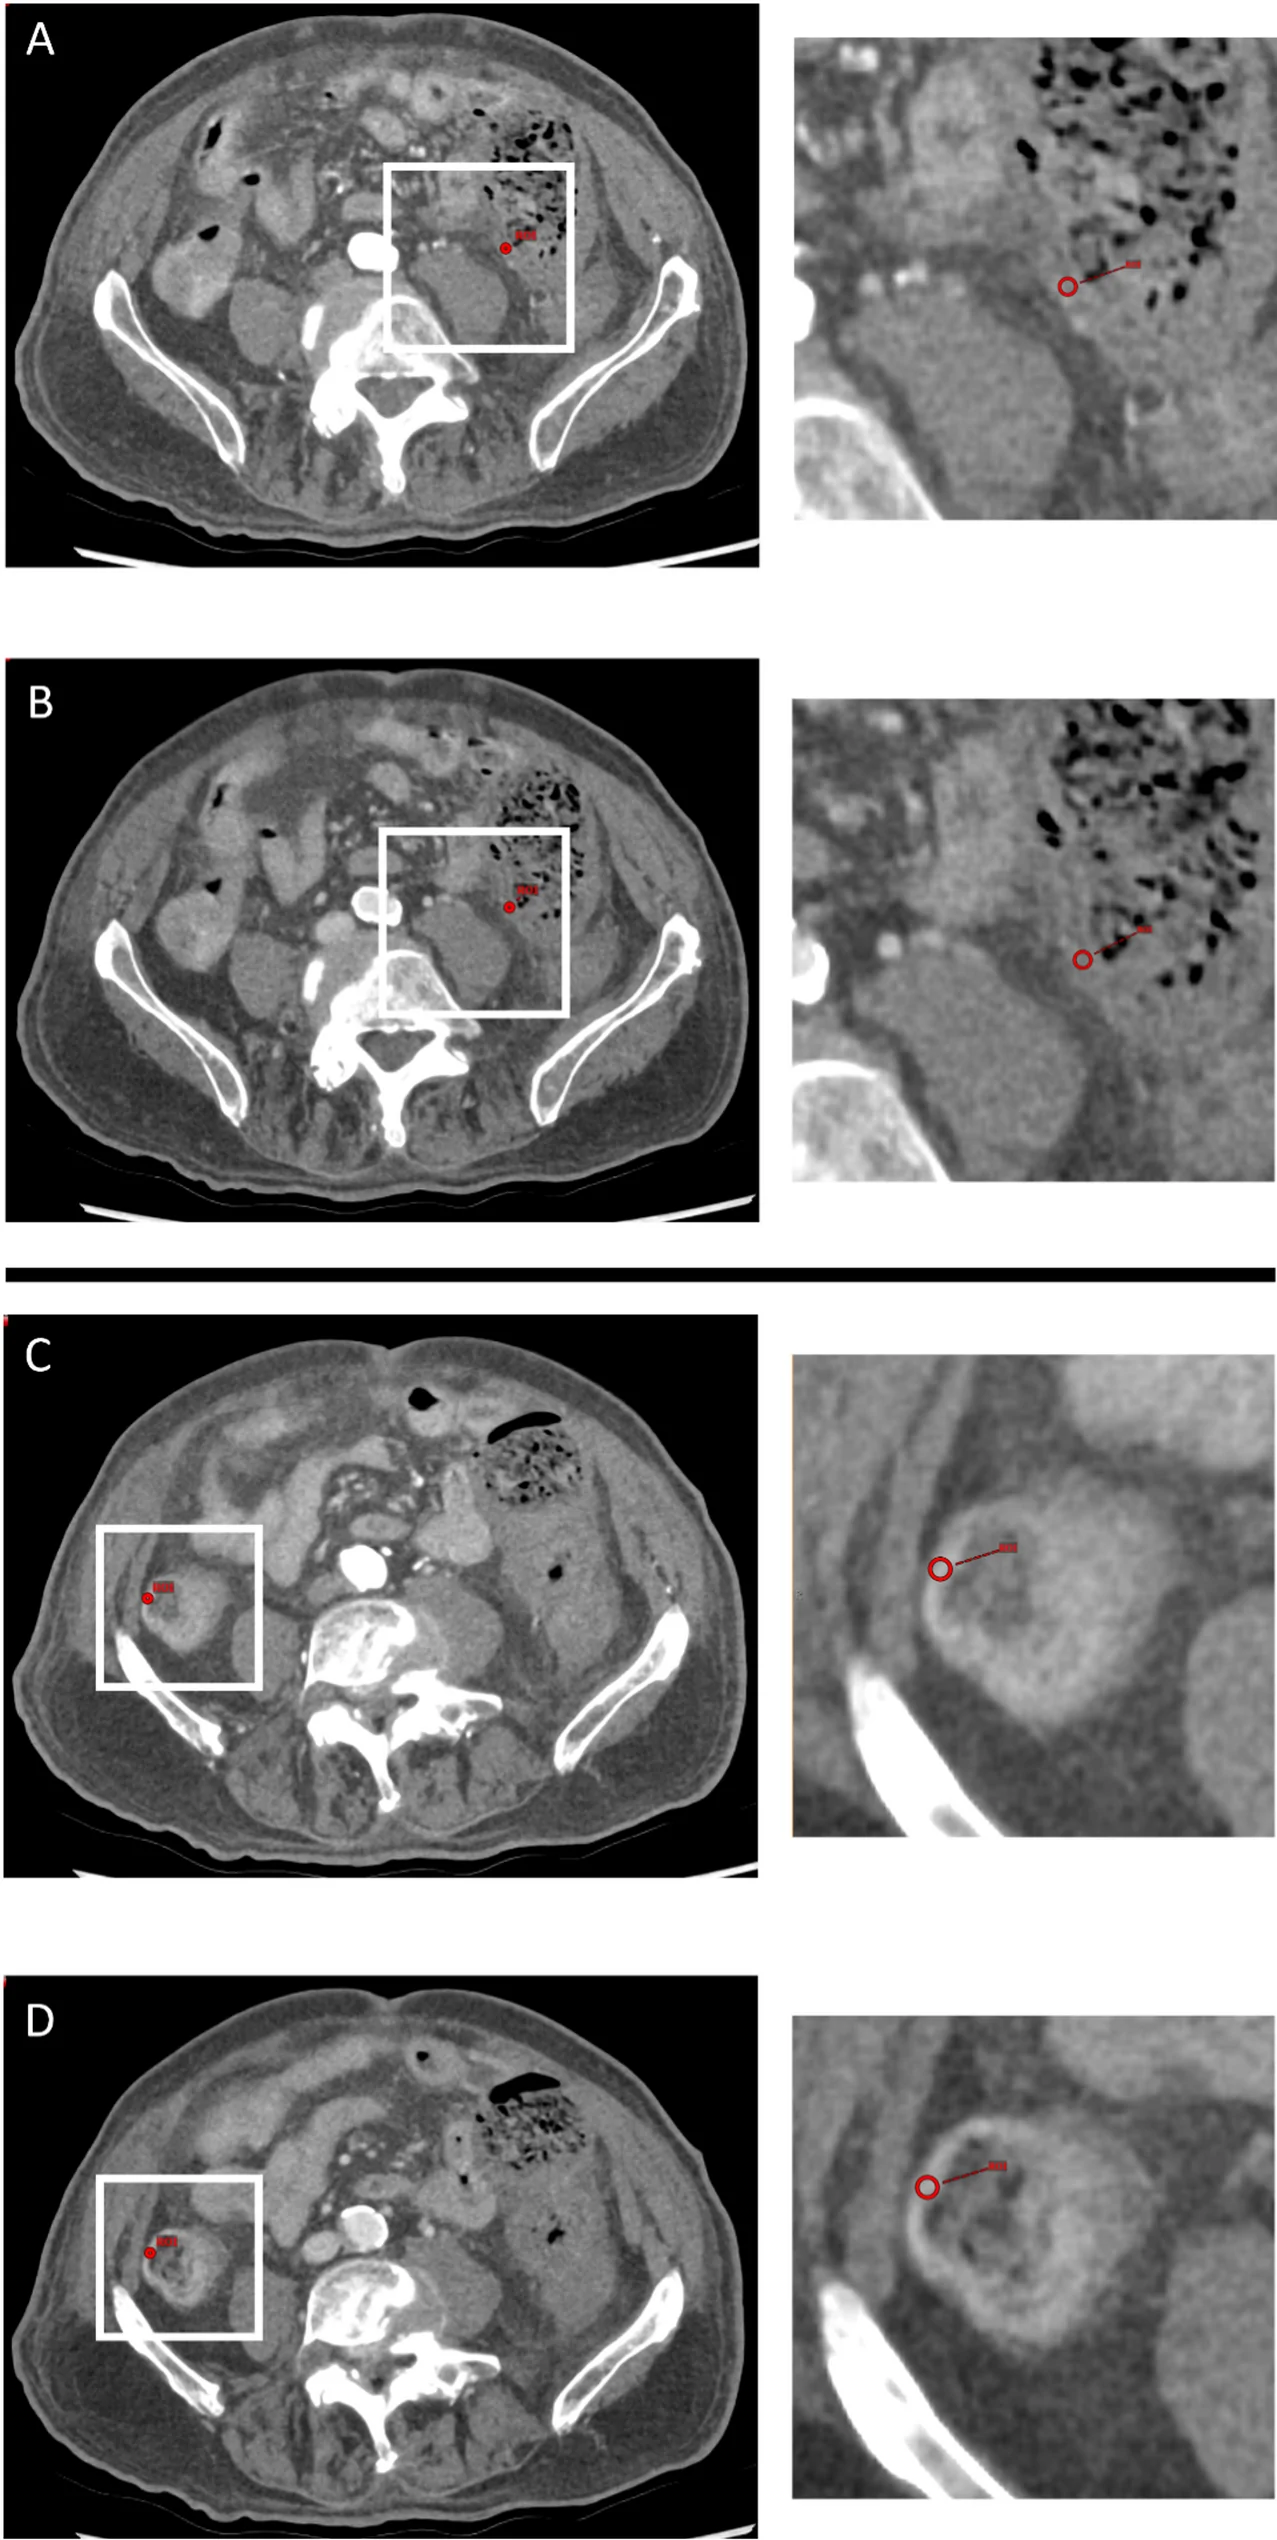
A-D: Analysis of bowel wall enhancement, placement of region of interest (ROI). Axial CT images of the abdomen with full field of view and zoomed selections (white box) of relevant bowel segments. 3 mm regions of interest (ROI, red) were placed in affected (A, B) and unaffected (C, D) bowel wall with identical placement in arterial (A, C) and venous (B, D) phase images
